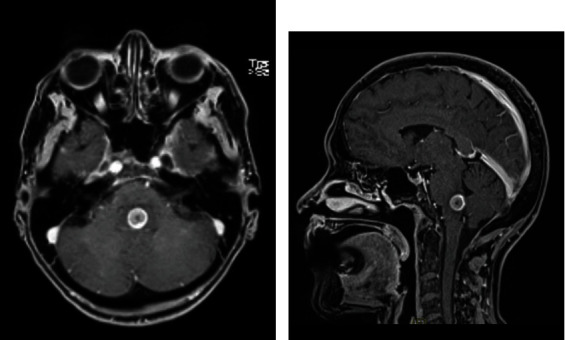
(a) Preoperative contrast-enhanced MRI shows a 13 mm intrinsic, roundish lesion in the posterior pontomedullary junction. (b) The floor of the inferior IV ventricle is slightly raised, and the tumor slightly protrudes from it.
